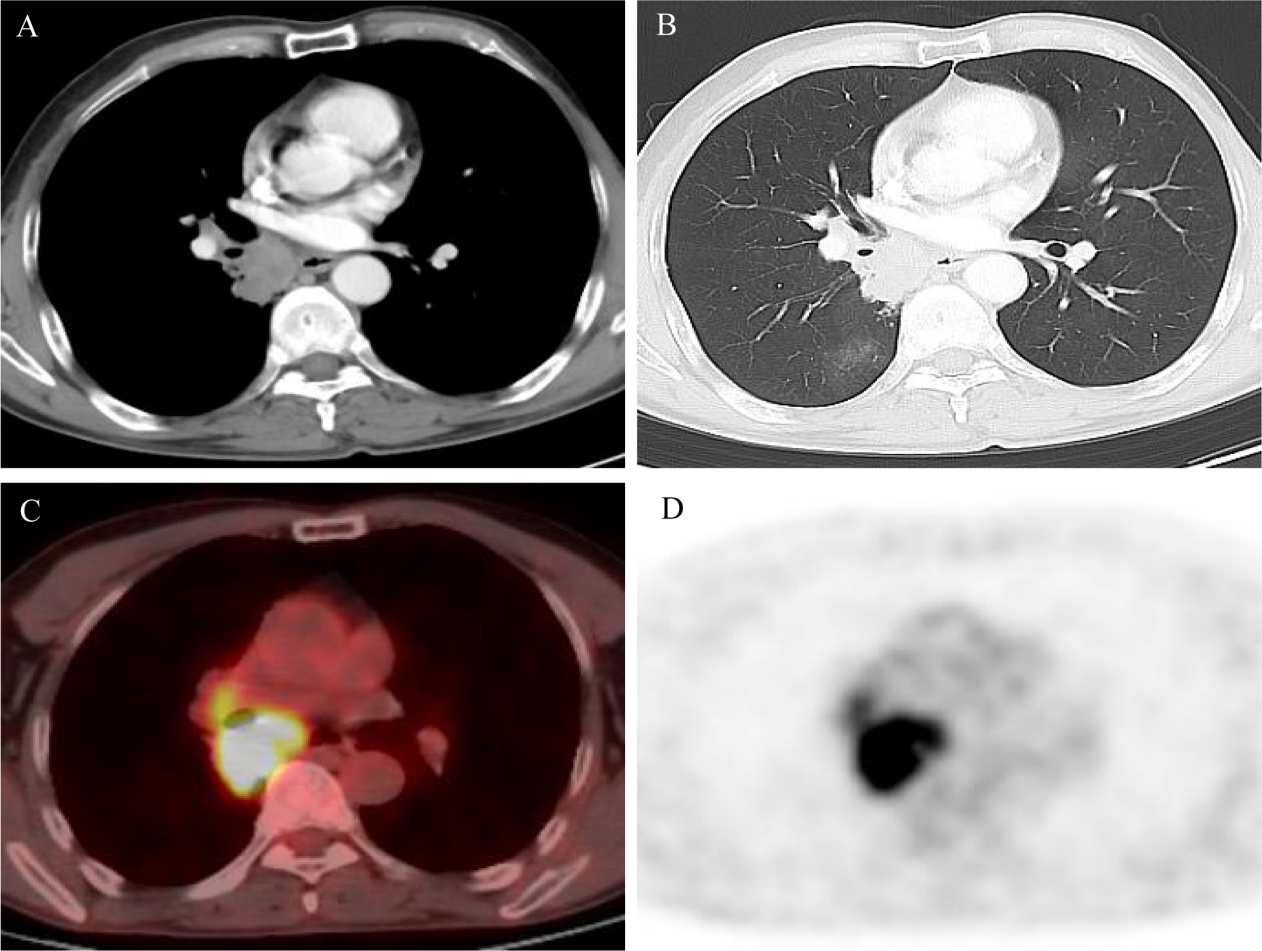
(A, B) Computed tomography (CT) scan at diagnosis showed a lung mass in the right lower lobe and mediastinum; (C, D) positron emission tomography/computed tomography (PET/CT) scan at diagnosis showed high fluorodeoxyglucose avidity.
